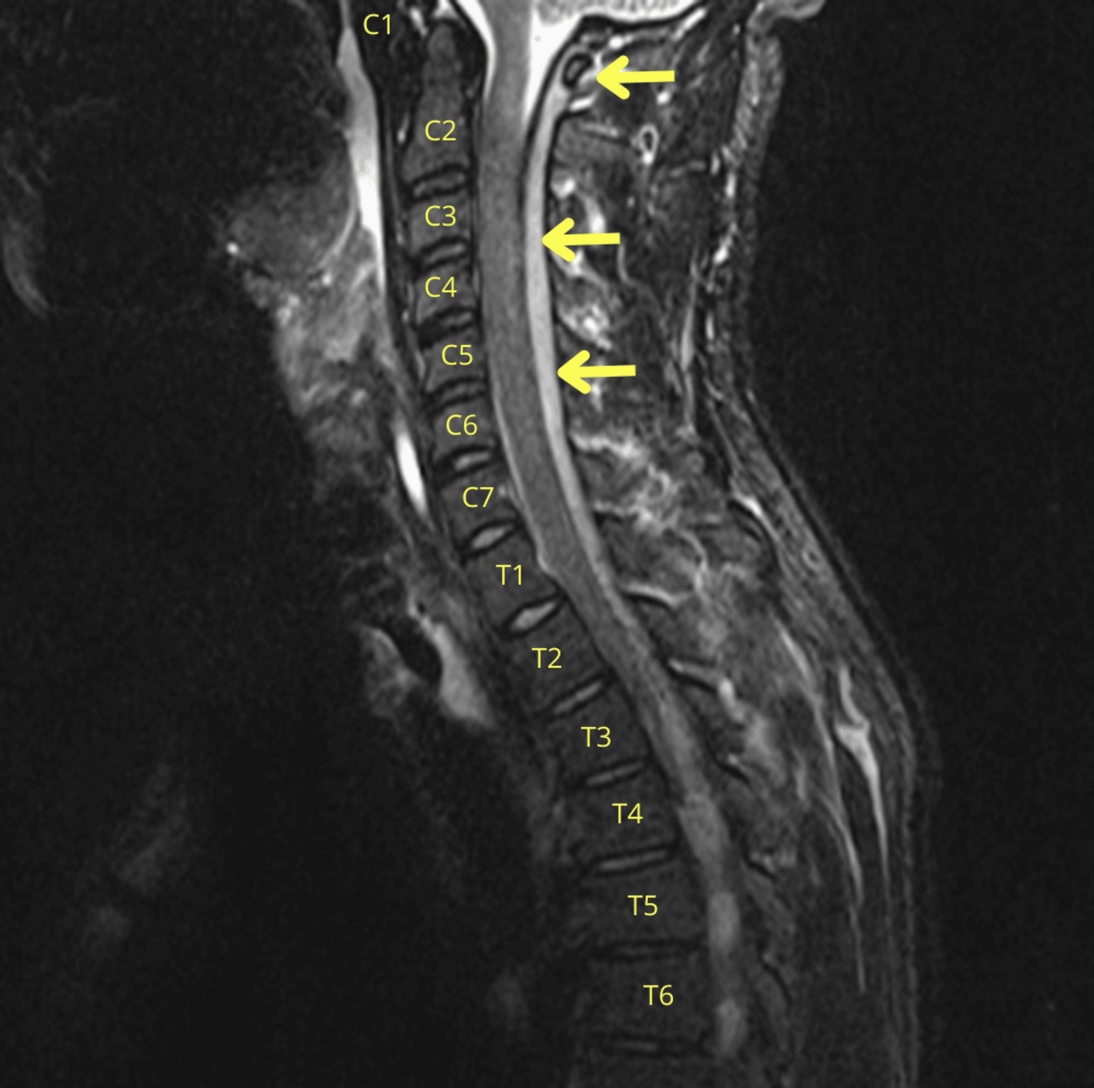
Sagittal cervical MRI scan T2-weighted images revealing extensive posterior-lateral epidural collection extending continuously from C1 to the sacrum (up to S2). Maximal thickness is ~6 mm at the cervical level (yellow arrows). MRI: magnetic resonance imaging
